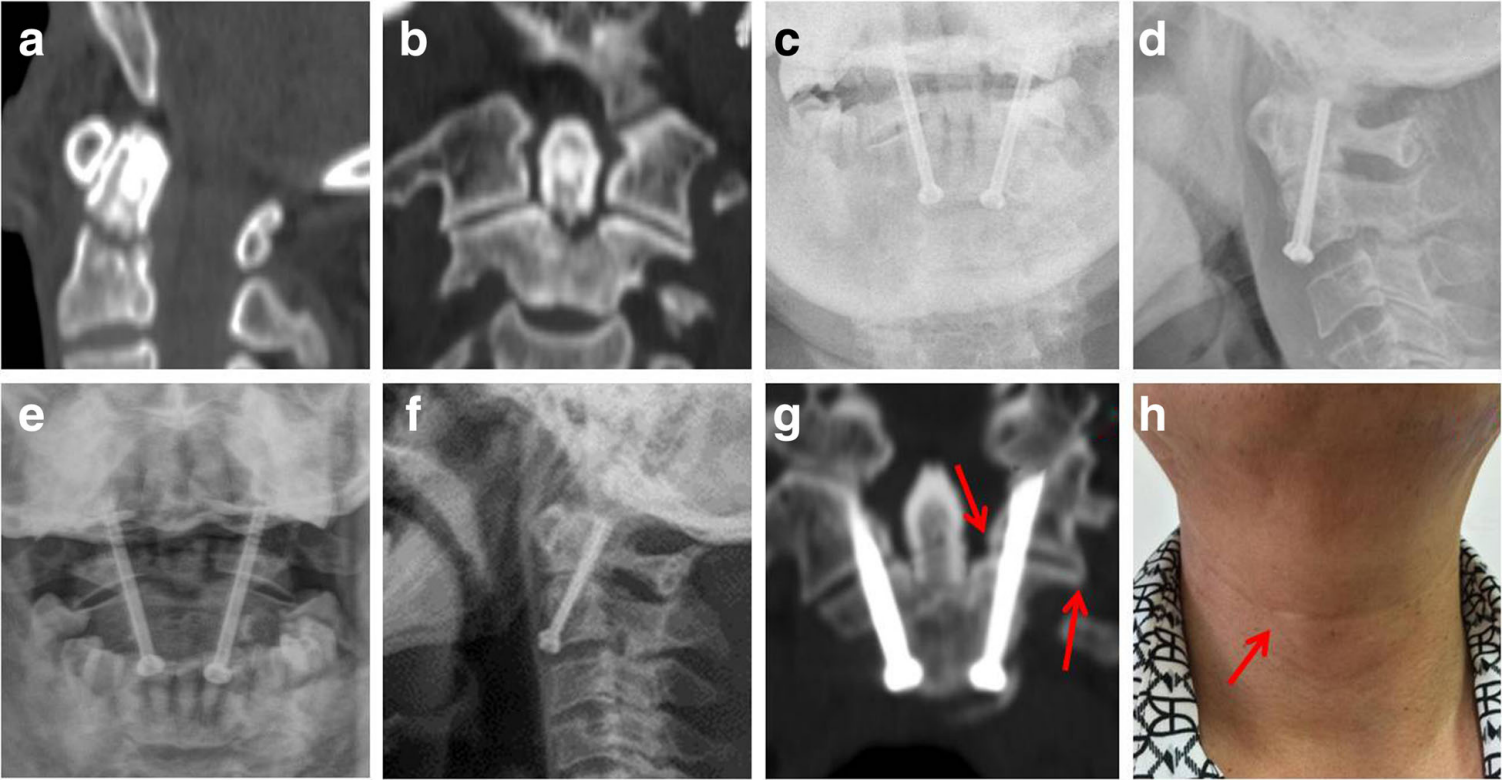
a, b The pre-operative 2D CT images of a 73-year-old man showed type II odontoid fracture. c, d Percutaneous anterior odontoid screw fixation was failed on him, and percutaneous C1/2 transarticular screw fixation was performed as salvage technique. e, f Thirty-eight months after operation, no screw loose or breakage was observed. g, h Radiographic bony fusion was observed at odontoid fracture site, and bony fusion bridge was observed at the C1/2 lateral articular facet (g, arrow), with only about 10-mm skin scar (h, arrow)
